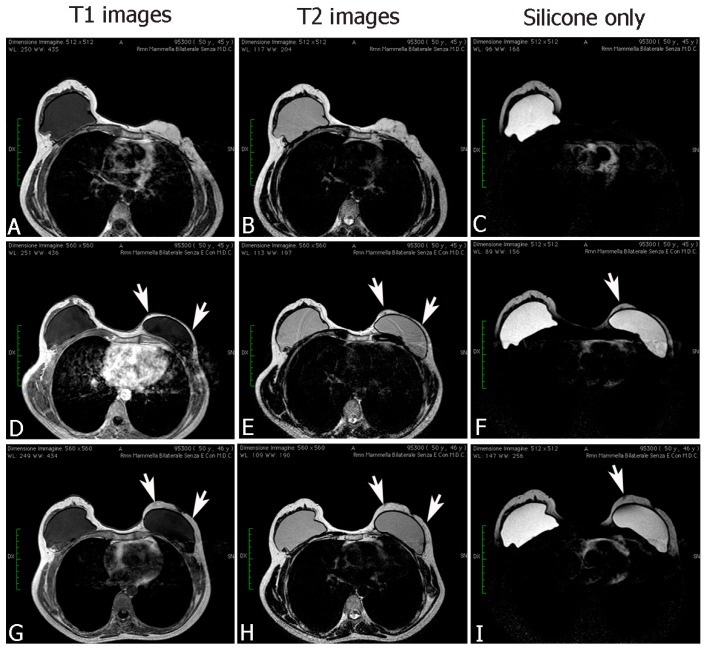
MRI figures, after fat transplantation to the breast, showed changes in volume, in thickness, and in fat distribution in the spaces (G–I) with respect to a previous treatment (D–F), and with respect to the pre-operative treatment (A–C). The fat grafting site was visible on MRI scans, with an identical signal of adjacent fat. The injected fat appearance is that of normal breast fat: hyperintense on T1- and T2-weighted sequences. On T1-weighted fat suppression images, the injected fat showed a hypointense normal fat signal. No signs of necrosis were presented on MRI.
